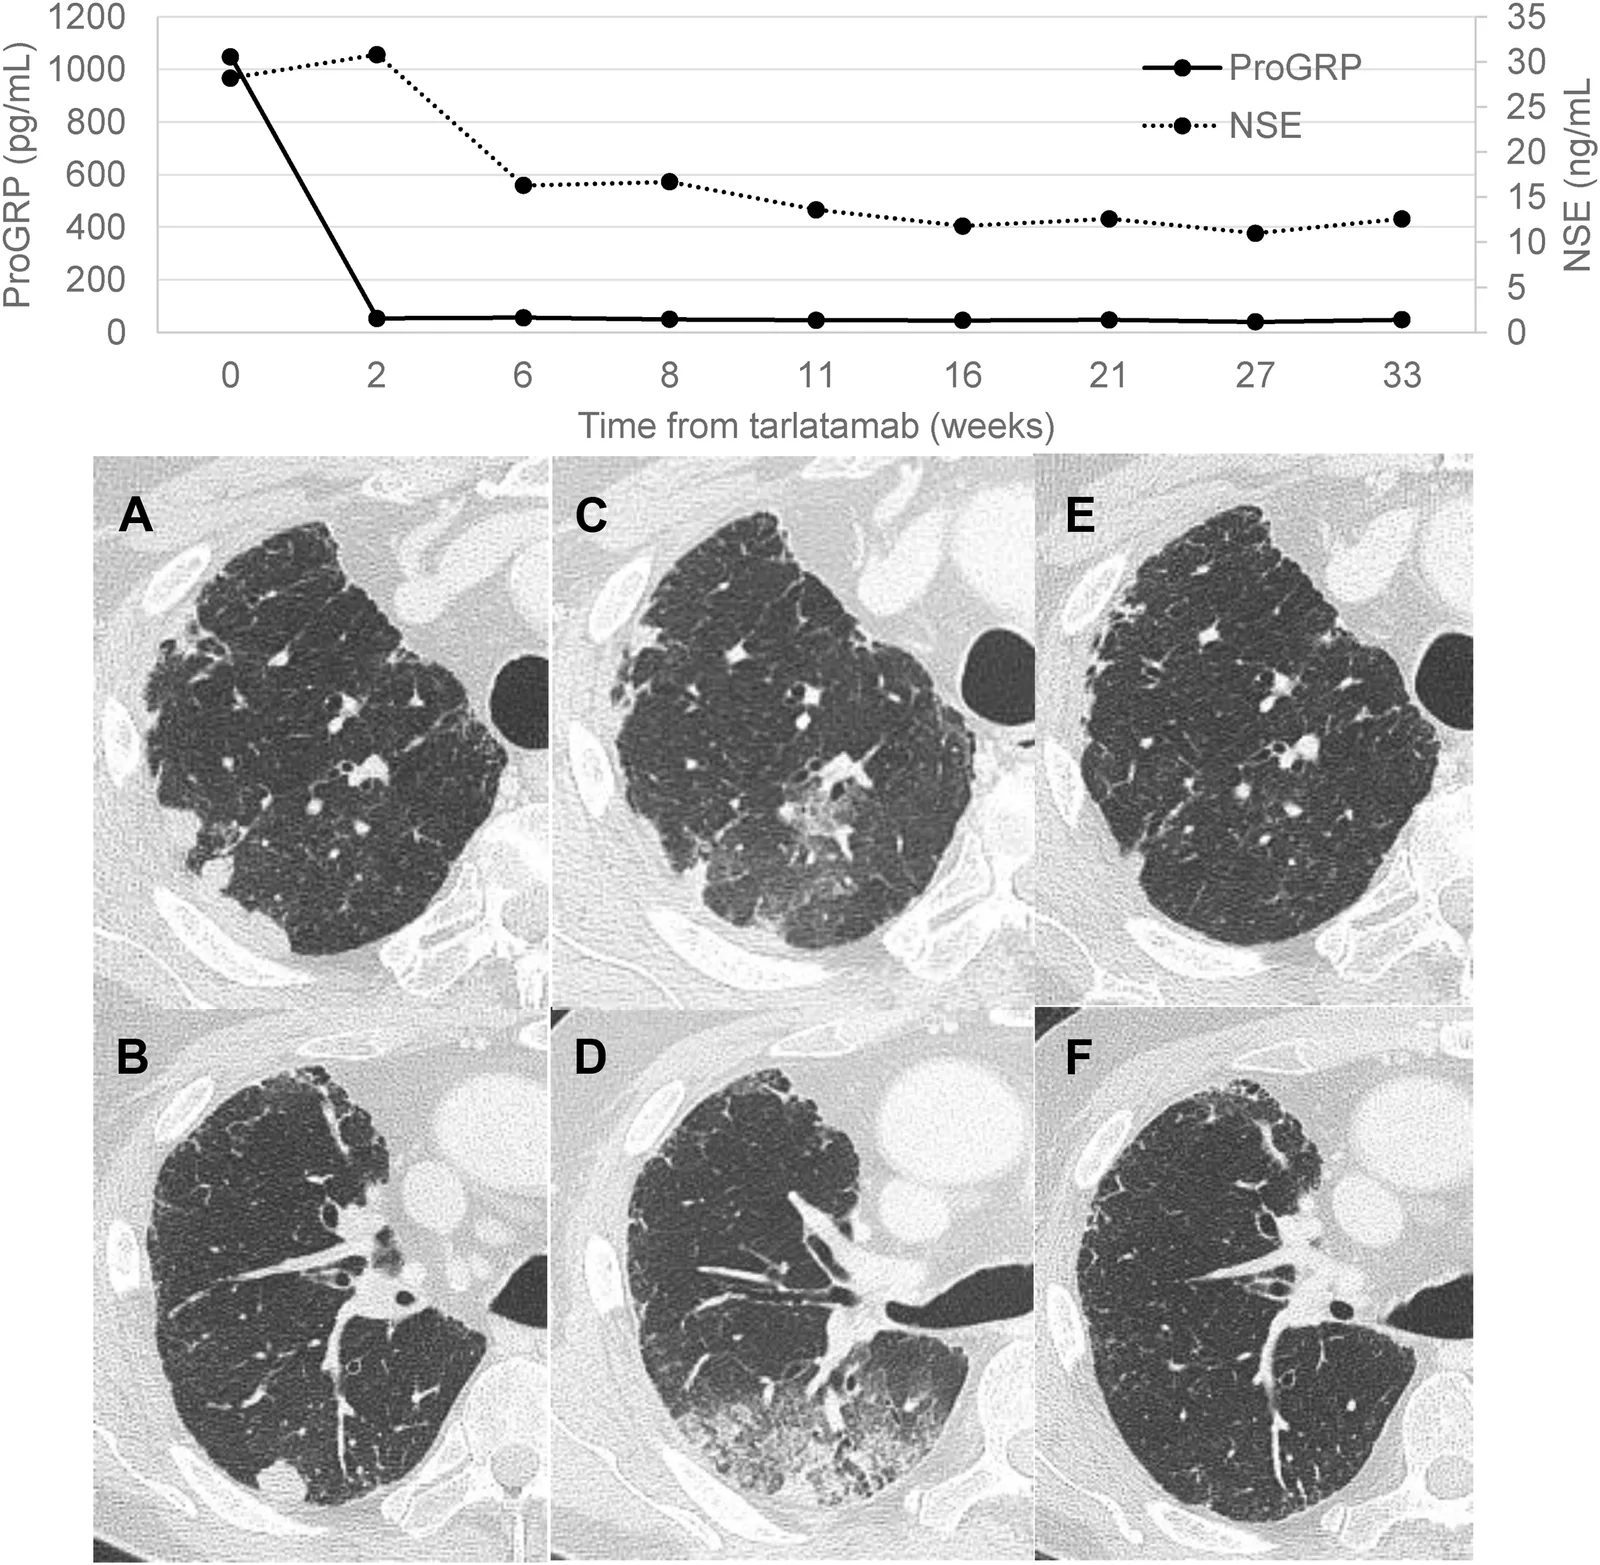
Treatment response to tarlatamab. Pretreatment HRCT reveals pleural dissemination and pulmonary metastases in the right lung (*A* and *B*). Two weeks after tarlatamab administration, HRCT reveals GGOs surrounding pulmonary metastases, accompanied by tumor shrinkage (*C* and *D*). After 33 weeks (8 mo) from tarlatamab administration, pleural dissemination in the right lung had resolved (*E* and *F*). Serum ProGRP and NSE levels normalized (upper limits of normal at our institution: ProGRP, 80.9 pg/mL; NSE, 16.3 ng/mL). GGO, ground-glass opacities; HRCT, high-resolution computed tomography; NSE, neuron-specific enolase; ProGRP, progastrin-releasing peptide.
